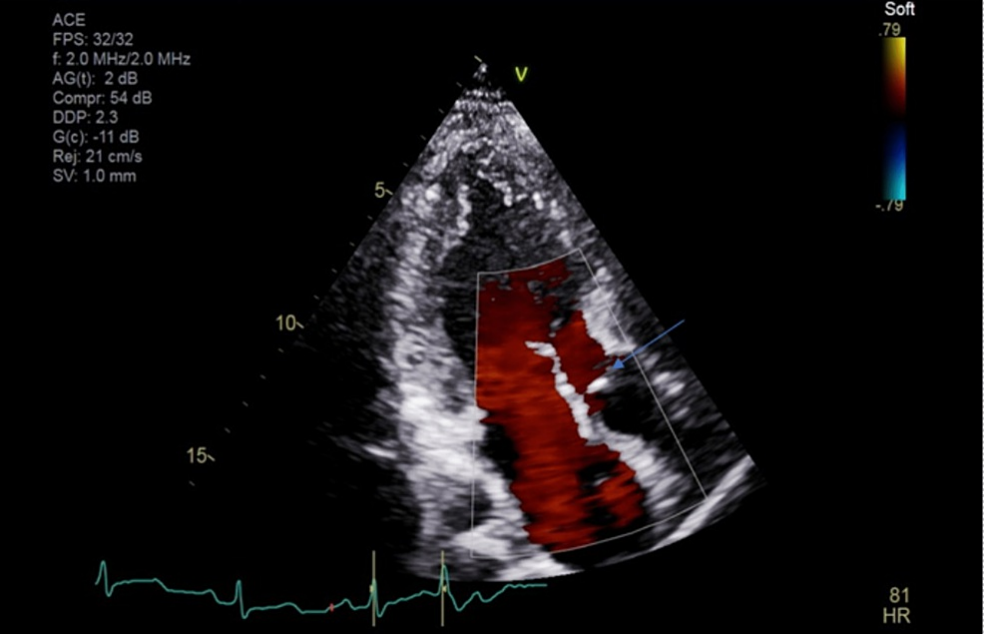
Echocardiogram with doppler – left Ventricular ejection fraction 55-60%. Subpulmonic VSD with Left to Right Shunt.
